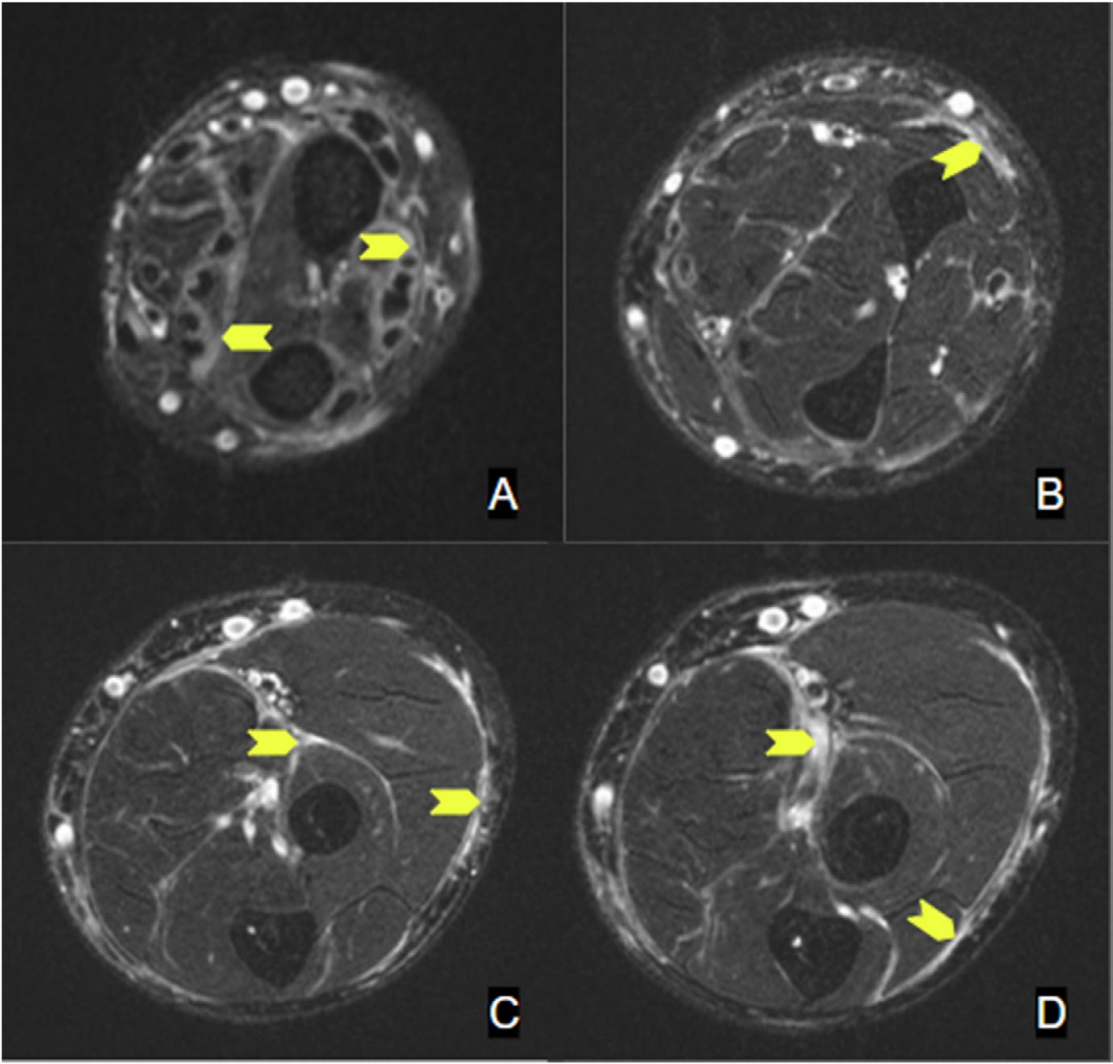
Left forearm axial T2‐weighted MRI. (A–D) distal to proximal demonstrating increased signal in the subcutaneous fascia.
